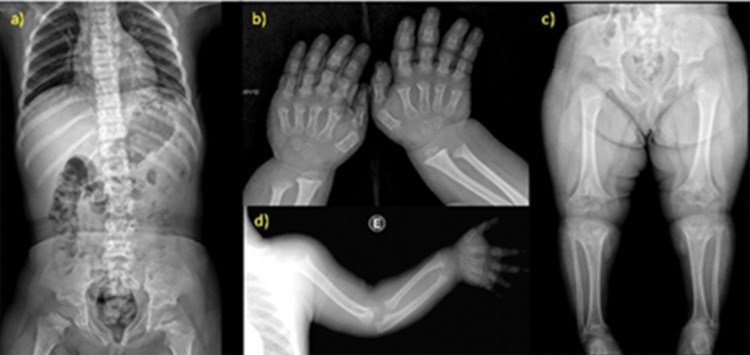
Radiological findings of achondroplasia. a-d) X-ray studies of a 5-year-old patient. a) Anterior view of the vertebral column shows narrowing of the interpediculate distance of the caudal spine; b) the typical hand presents brachydactyly with a trident configuration; c) the pelvis has rounded ilia and horizonal acetabula, and the femurs are short with proximal radiolucency; d) the short humerus also demonstrates rhizomelic dwarfism.
